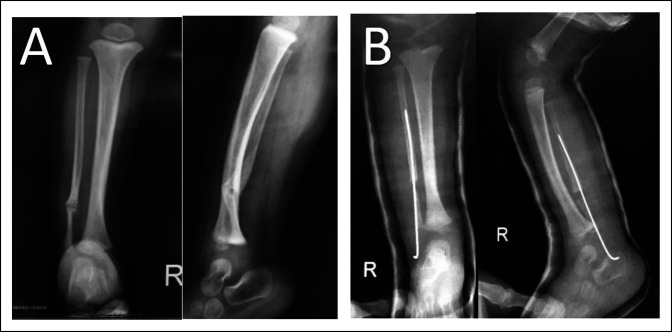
A 9-month-old boy with congenital pseudarthrosis (congenital pseudarthrosis of the fibula) of the fibula type 3 Dooley (A), as seen in the postoperative radiograph. A transferred periosteal flap technique was conducted for the patient (B).
